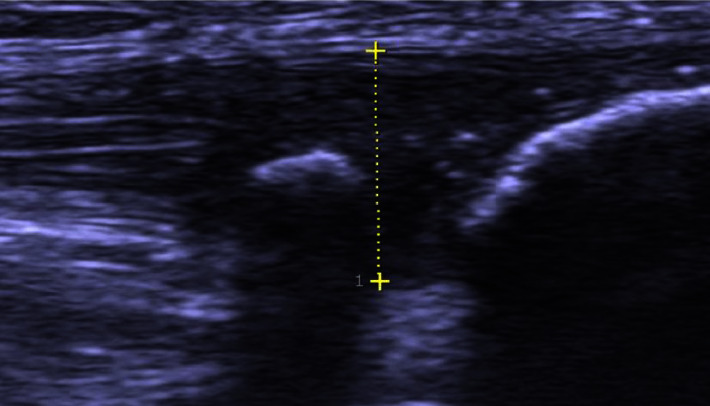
Grey scale ultrasound examination-longitudinal view on a patient with proximal patellar tendinopathy. There is a thickening of the proximal patellar tendon (marked) including tendinopathy on the dorsal side of the tendon, a loose intratendinous bone fragment and an indication of a possibly sharp bone edge in the patellar tip.
